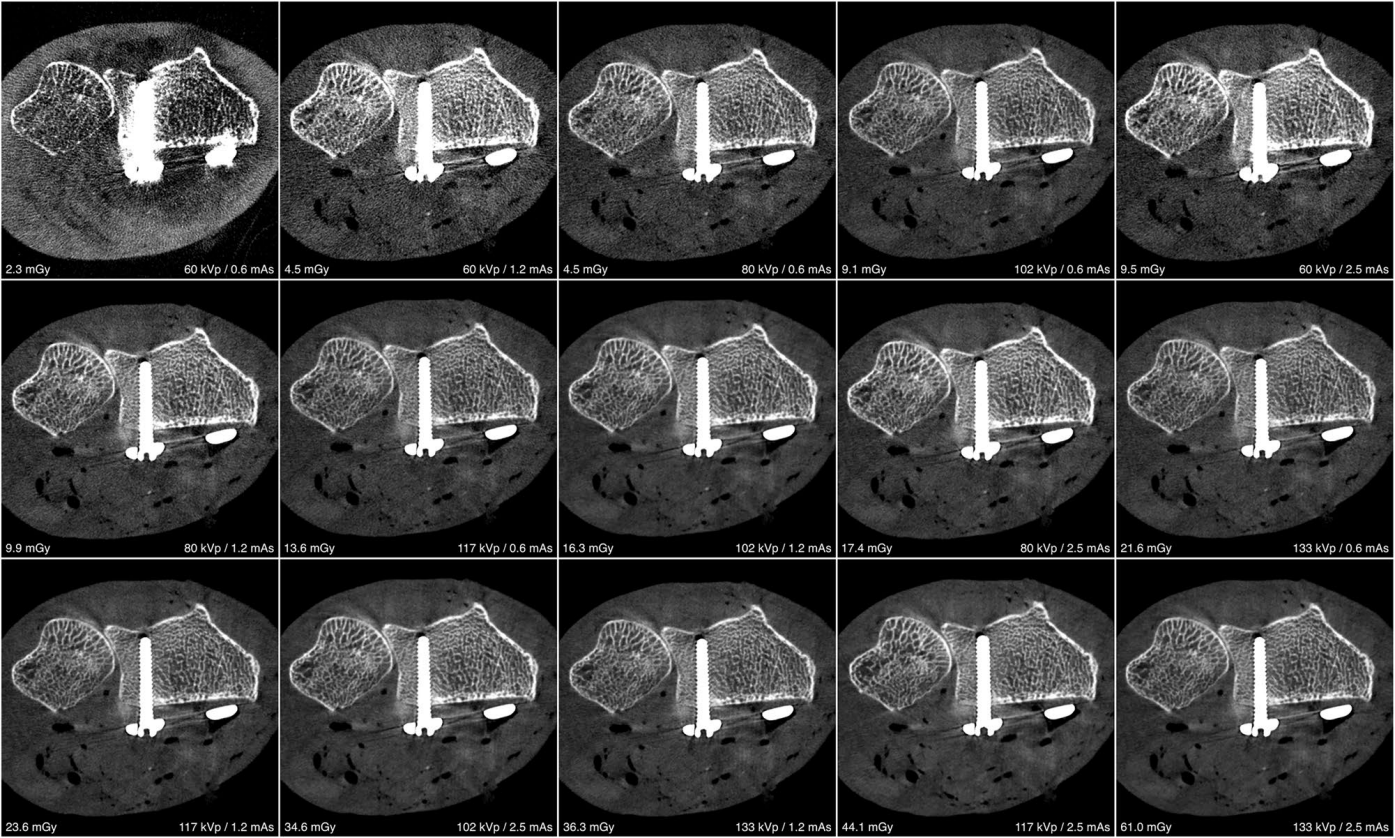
Bone image quality and intensity of metal artifacts by palmar plate osteosynthesis in ultra-high-resolution CBCT scan protocols.
